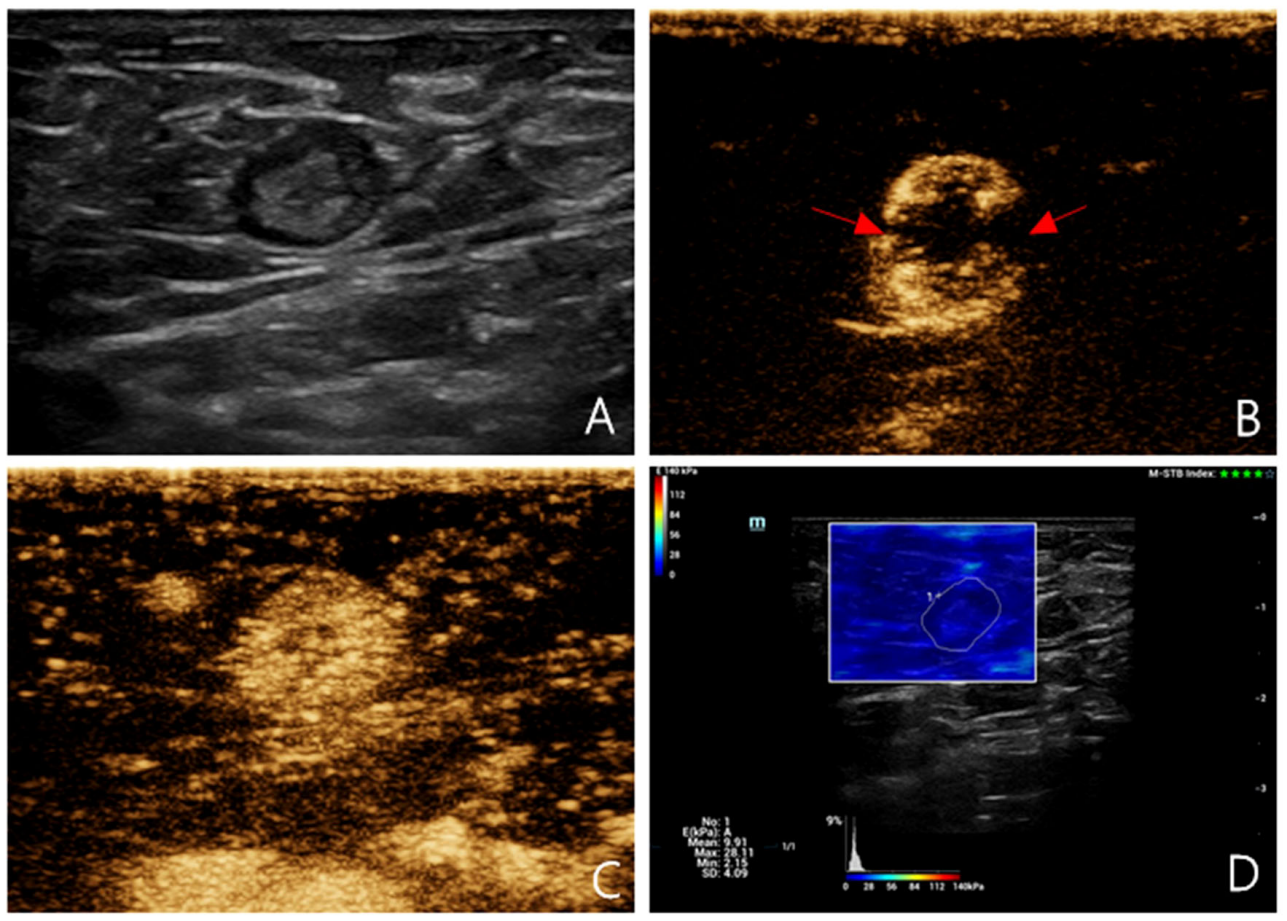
Multimodal ultrasound imaging of pathologically confirmed non-metastatic sentinel lymph node. (A) 2D US image; (B) Intradermal CEUS image (the enhancement pattern is type IV, the red arrows indicate local perfusion defect in the SLN); (C) Intravenous CEUS image (the enhancement pattern is mixed); (D) SWE image (Emean=9.91 kPa).
